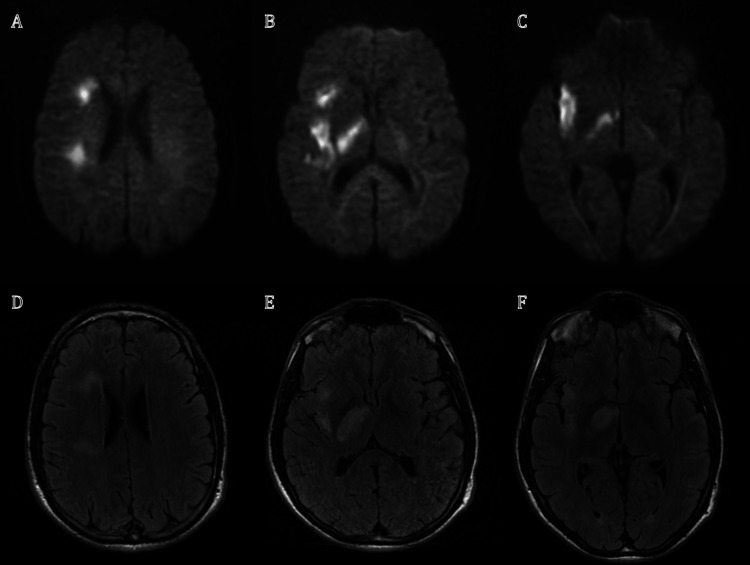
MRI study from patient 2 Panels A-B-C: DWI sequence showing hyperintense signal in the right internal, external capsules (A-B) and in the deep white matter of the frontal lobe (C). Panels D-E-F: Corresponding FLAIR sequences showing the extension of the ischemic lesion.
